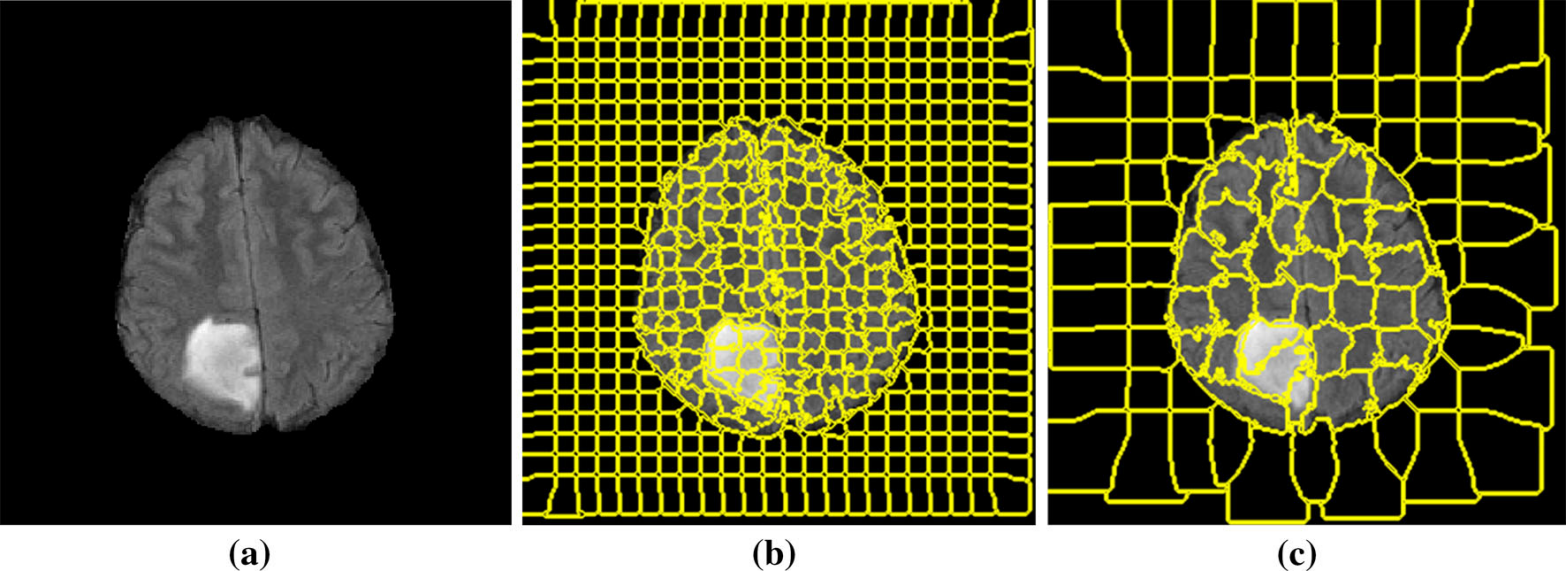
Example of superpixel segmentation with different window sizes: a original MRI FLAIR image with a grade II tumour, b superpixel segmentation with $$S= 10$$ (initial grids $$10\times 10$$) and $$m = 0.2$$, c superpixel segmentation with $$S = 20$$ (initial grids $$20\times 20$$) and $$m = 0.2$$
